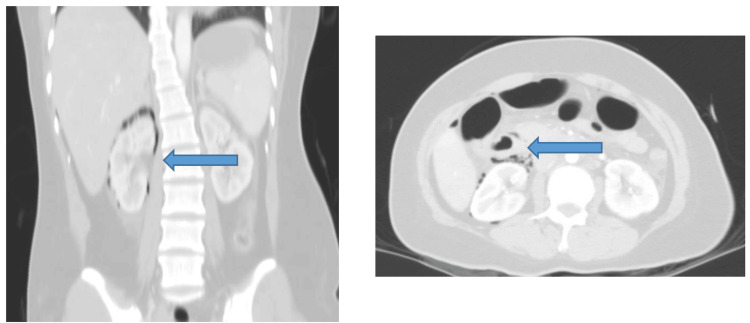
CT showed large volumes of free intraperitoneal air, predominantly around the pancreatic head and duodenum.
